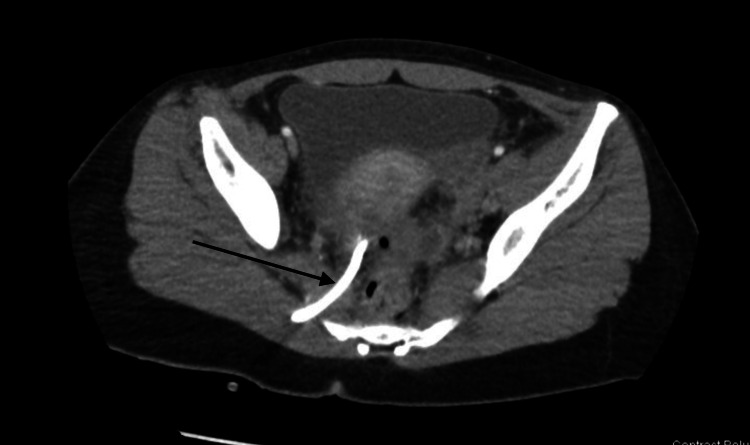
CT abdomen/pelvis The image is showing resolution of the fluid collection with the presence of a drainage catheter (arrow).
